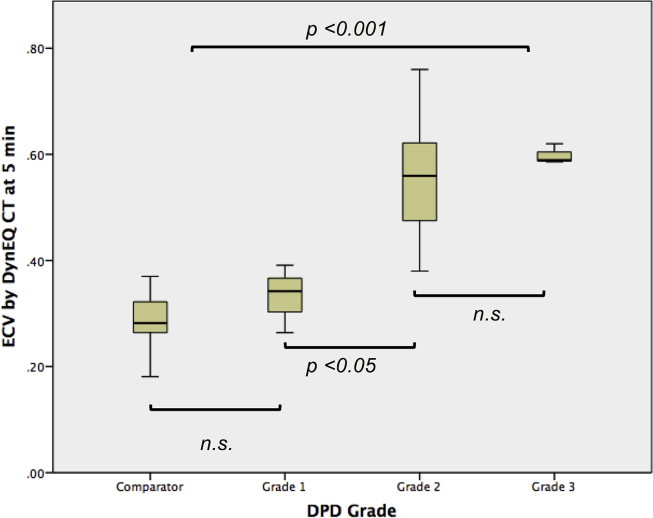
ECV tracks amyloid burden measured by DPD bone scintigraphy: ECV vs DPD grade in 26 patients with systemic amyloidosis (27 patients with aortic stenosis used as comparator – no evidence of cardiac involvement on myocardial biopsy).
